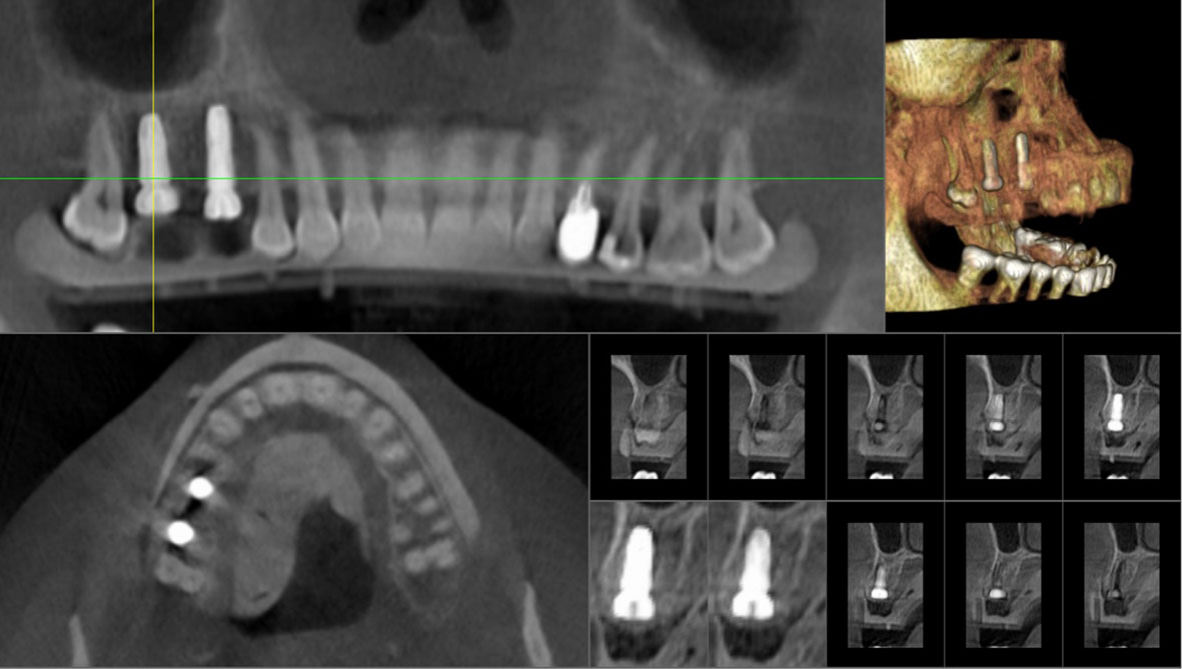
Cone beam computed tomography (CBCT) for the control of implant placement
